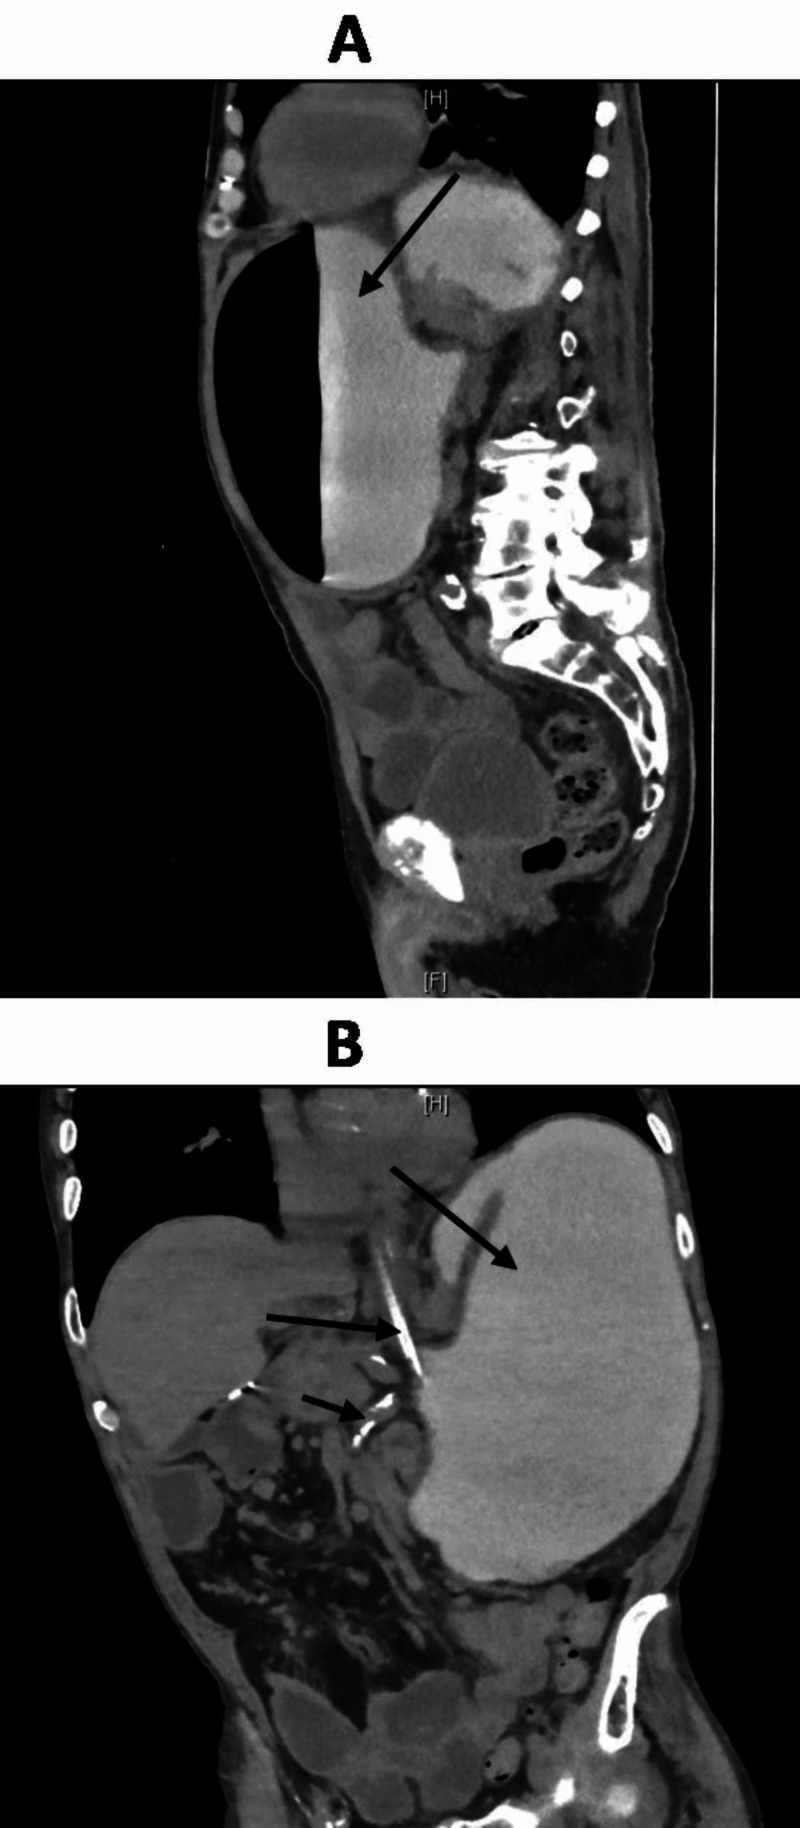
Coronal (A) and sagittal (B) views of CT abdomen with oral contrast Findings are consistent with a mesenteroaxial orientation or incomplete mesenteroaxial gastric volvulus with some of the contrast material passing into the duodenum and proximal jejunal loops. The focal short segment is seen narrowing at the level of the junction of the gastric antrum/duodenum. Contrast material is seen within the partially opacified stomach. In addition, it is important to note that the contrast material is passing into the duodenum and proximal jejunal loops consistent with partial obstruction secondary to the focal narrowing at the level of the gastric antrum/proximal duodenum CT: computed tomography
